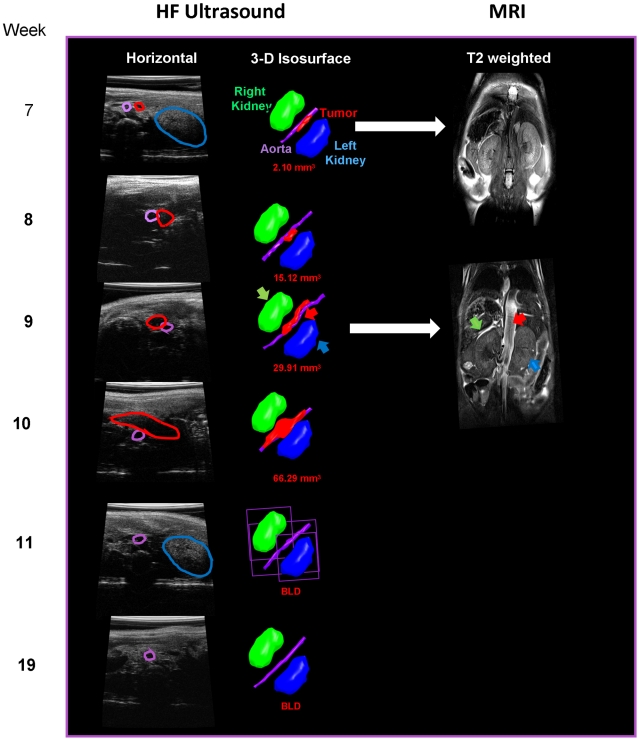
Monitoring of regressing TH-MYCN tumors by imaging. Regressing TH-MYCN tumors were imaged by ultrasound and MRI between weeks
